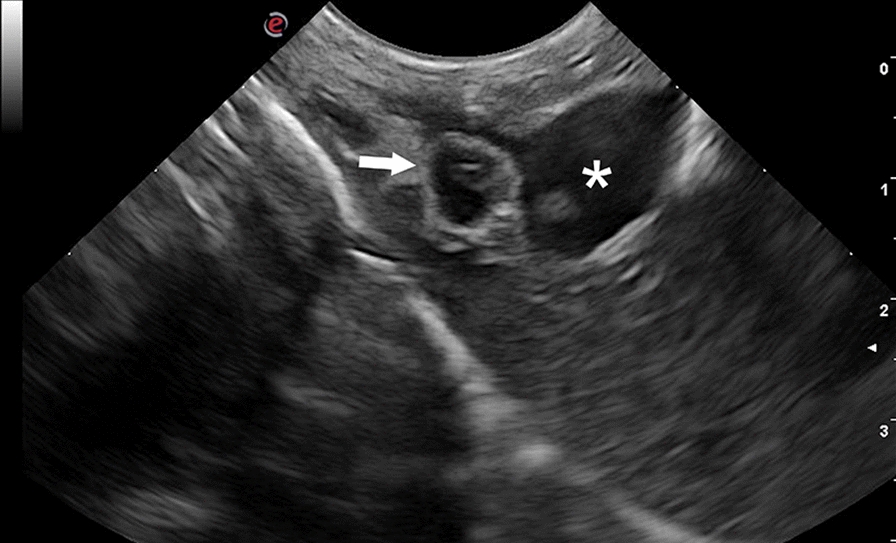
Ultrasonographic longitudinal section of the liver showing a cystic structure containing a hyperechoic mural branching component (arrow) localized along the hepatic visceral surface at the level of the portal vein (asterisk)
